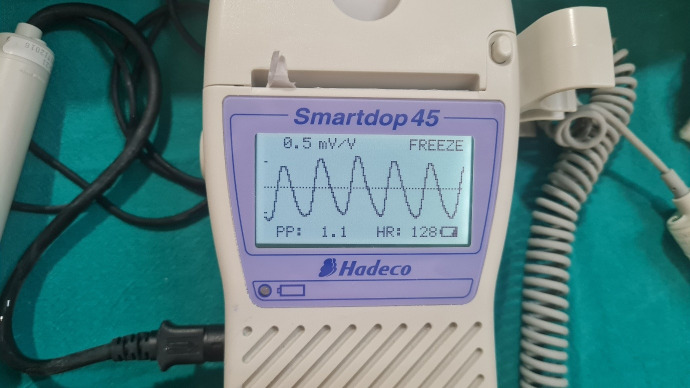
Appearance of photoplethysmography waveform in Doppler
